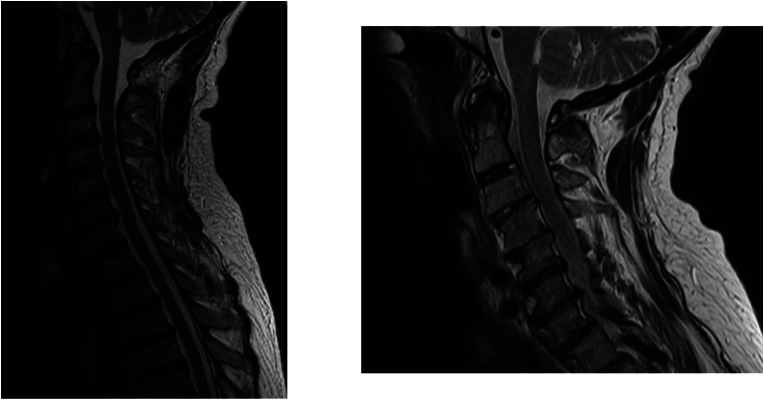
Patient who progressed into a case during FU. On the FU image (right) pannus can be observed, as well as dens erosions. These sagittal T2-weighted MRI images show the progression of a patient from a clean baseline scan to pannus formation and dens erosions on the follow-up scan.
